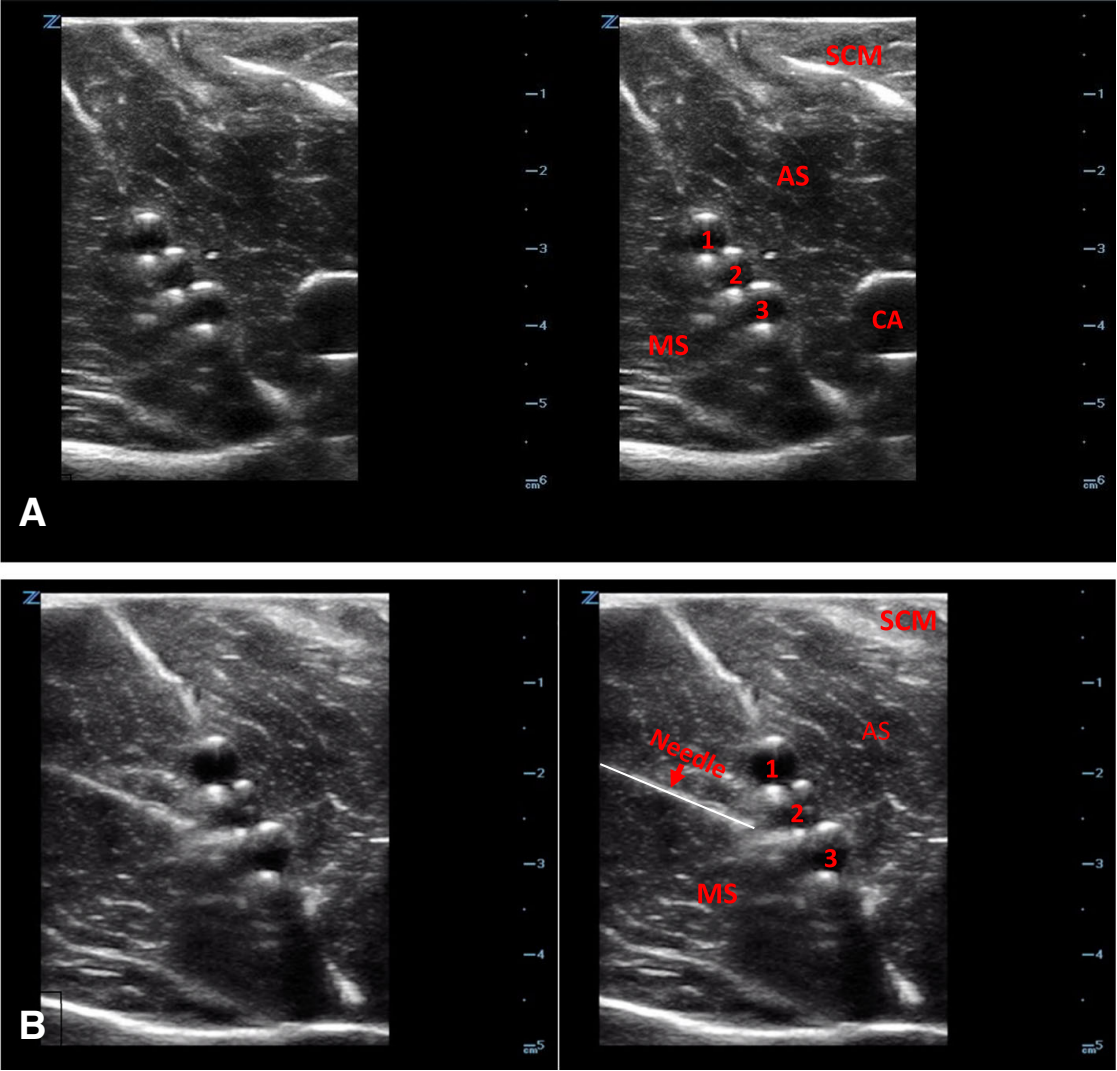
Ultrasound image of the interscalene brachial plexus model (a). Ultrasound image of needle placement (b). SCM, sternocleidomastoid muscle; AS, anterior scalene muscle; MS, middle scalene muscle; CA, carotid artery; IS, interscalene plexus; 1, C5 nerve root; 2, C6 nerve root; 3, C7 nerve root
